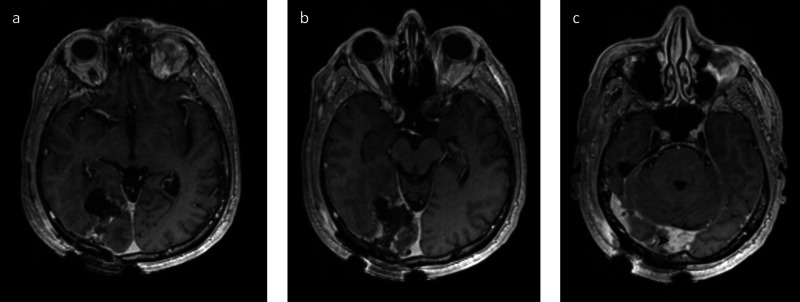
Postoperative MRI Postoperative T1-weighted post-contrast MP-RAGE images along the super (a), mid (b), and inferior (c) aspects of the resection cavity. The majority of the resection cavity exhibits thin peripheral post-contrast enhancement, likely post-surgical in nature. However, along the inferior aspect of the resection cavity, where the tumor was previously seen to involve the straight sinus, and torcula, there is a residual thickened enhancement (arrowhead) concerning for residual tumor, corresponding to an unresected tumor on the operative report MRI: magnetic resonance imaging; MP-RAGE: magnetization-prepared rapid gradient-echo
